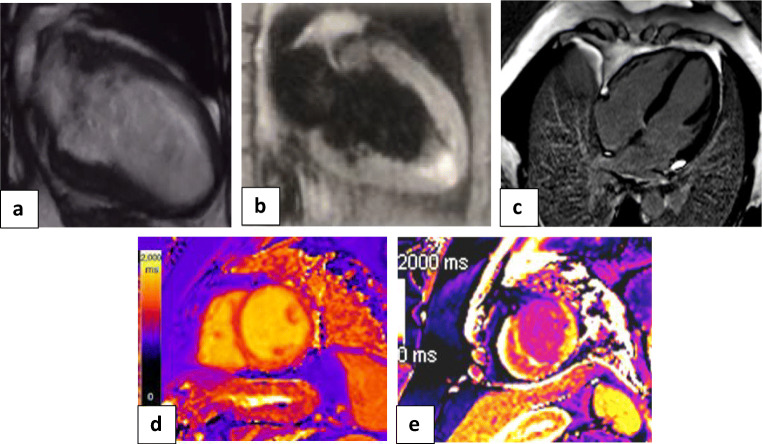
Cardiac MRI: a white blood image 2-chamber vertical long-axis view showing ballooning of the cardiac apex. b T2-weighted-STIR image 2-chamber view showing interstitial edema of the cardiac apex. c IR post-contrast image 4-chamber view showing no appreciable LGE of the cardiac apex. d, e T2 and T1 cardiac MRI mapping sequence in two-chamber views respectively showing intense interstitial myocardial edema (native T2 = 64 ms) and elevated T1 value =1178 ms. Conventional coronary angiography of LAD confirmed normal appearance with no stenosis or occlusion. The final diagnosis was Takotsubo-like cardiomyopathy (as a part of acute myocarditis COVID-like syndrome (AMCovS)
